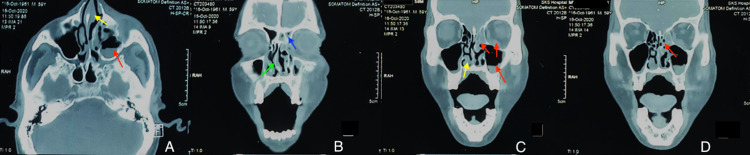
MDCT axial section (A) and coronal section (B-D) of facial bones. Nasal mucosal thickening (yellow arrow), mucosal thickening in left frontal sinus (blue arrow), mucosal thickening and fluid in the left maxillary, ethmoidal sinus and inflamed inferior rectus (red arrow), and deviation of nasal septum ( green arrow). MDCT: Multidetector computed tomography
